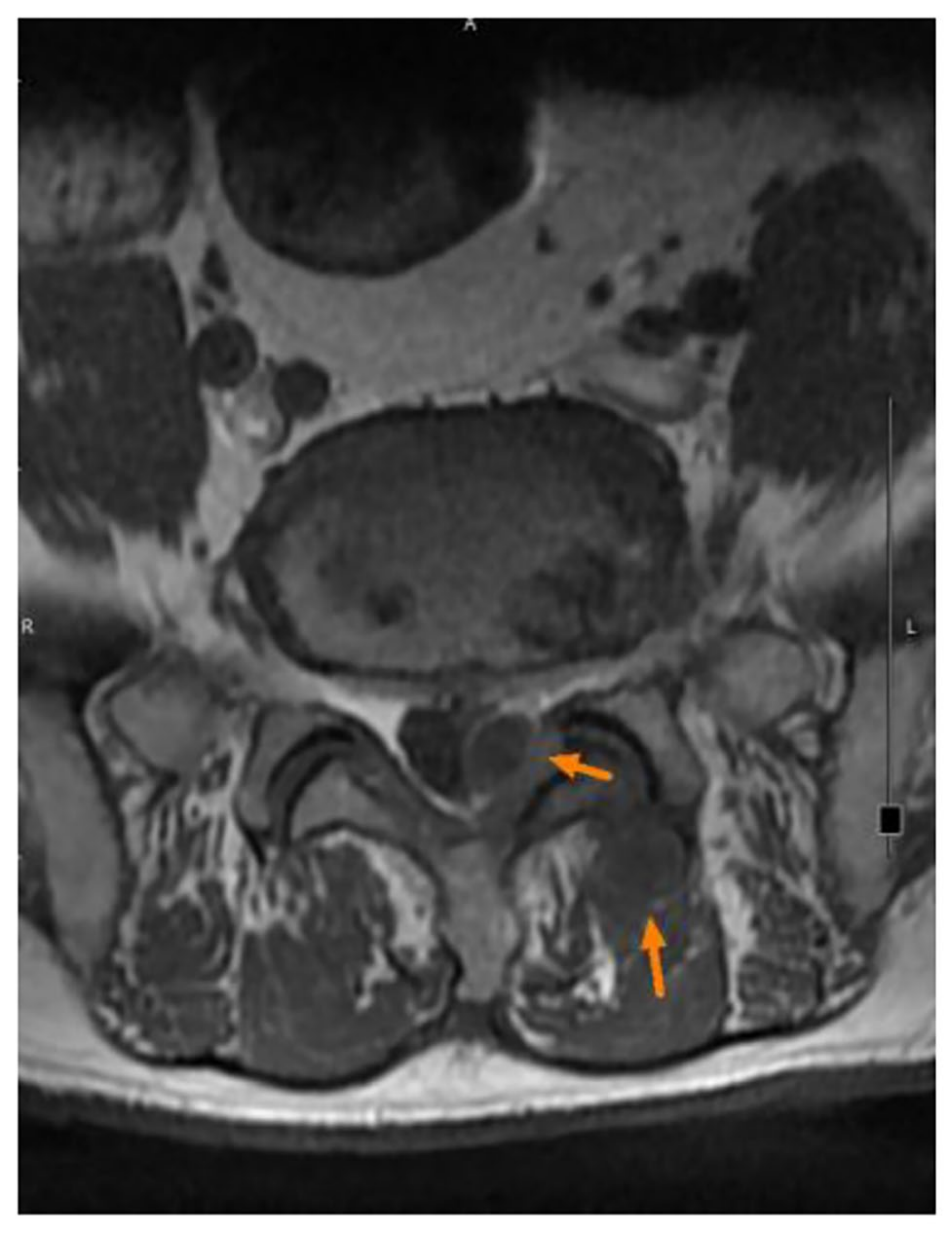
Shows the hypointense signal of the masses in the T1-weighted image
